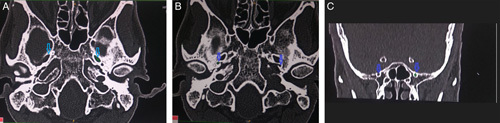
(A) Axial computed tomography image of the skull base (bone window) showing a method of measurement of foramen ovale (arrows), with calipers placed on length and width of left foramen ovale (solid lines). (B) Axial computed tomography image of the skull base (bone window) showing a method of measurement of foramen spinosum (arrows), with calipers placed on length and width of right foramen spinosum (solid lines). (C) Reformatted coronal computed tomography image of the skull base (bone window) showing a method of measurement of foramen rotundum (arrows), with calipers placed on the height and width of left foramen rotundum (solid lines).
